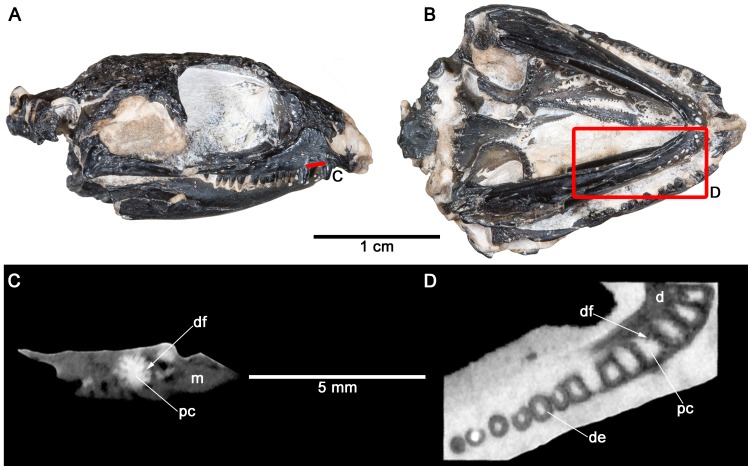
Cross-sectional views of the maxillary and dentary dentition of *Feeserpeton oklahomensis* (OMNH 73541) obtained via X-ray computed tomography (CT) scans. A: skull of *F. oklahomensis* in right lateral view showing from where the virtual sections of the maxilla were taken. B: skull of *F. oklahomensis* in ventral view showing from where the virtual sections of the dentary were taken. C: virtual cross-section of the base of one of the enlarged maxillary teeth showing the presence of tight dentine infolding. D: virtual cross-section of the bases of the dentary teeth showing the presence of dentine infolding. d, dentary; de, dentine; df, dentine fold; m, maxilla; pc, pulp cavity.
